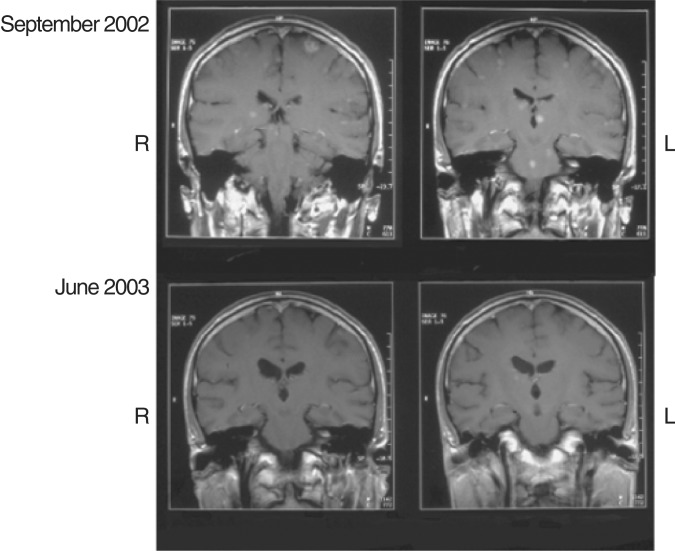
Computed tomography scan before and after treatment with gefitinib and radiotherapy (case history shown in Figure 2). Previously presented by: van Zandwijk N. Risk/benefit of gefitinib (‘Iressa’, ZD1839). Oral presentation at the ICE meeting, Madrid, June 2003. Reproduced with permission.
